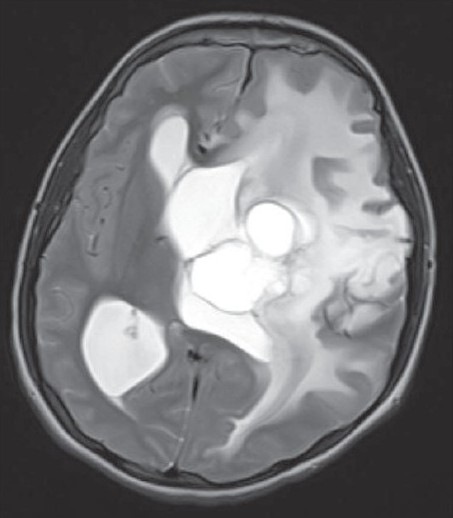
Axial section of the T2-weighted magnetic resonance imaging image of the brain showing solid and cystic components and peritumoral edema
